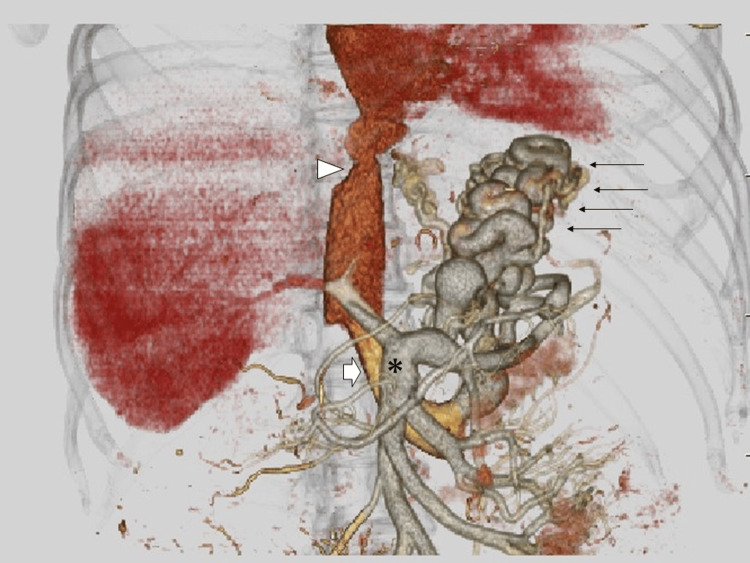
Postoperative contrast-enhanced CT A catheter was placed in the superior mesenteric artery (SMA), followed by injection of a contrast agent and subsequent CT imaging. The scan shows inferior vena cava (IVC) stenosis (▷), renal vein (⇨), gastrorenal shunt (→), and superior mesenteric vein (＊).
